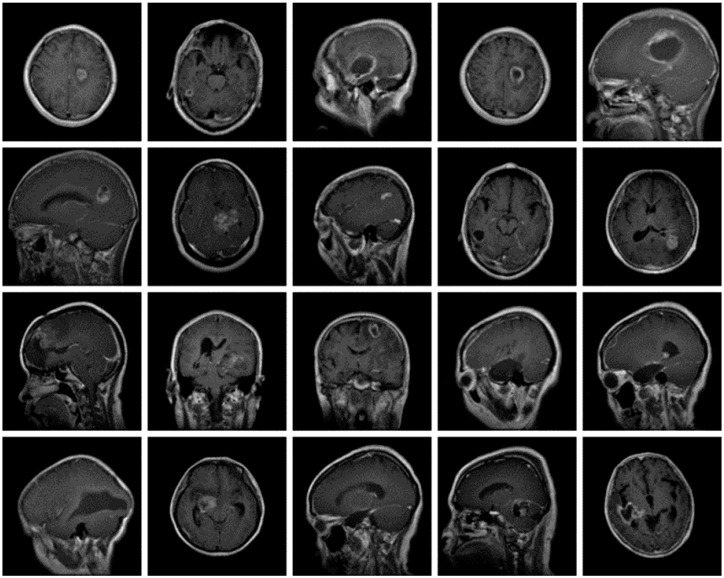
Examples of MRI scans of Glioma tumors.
